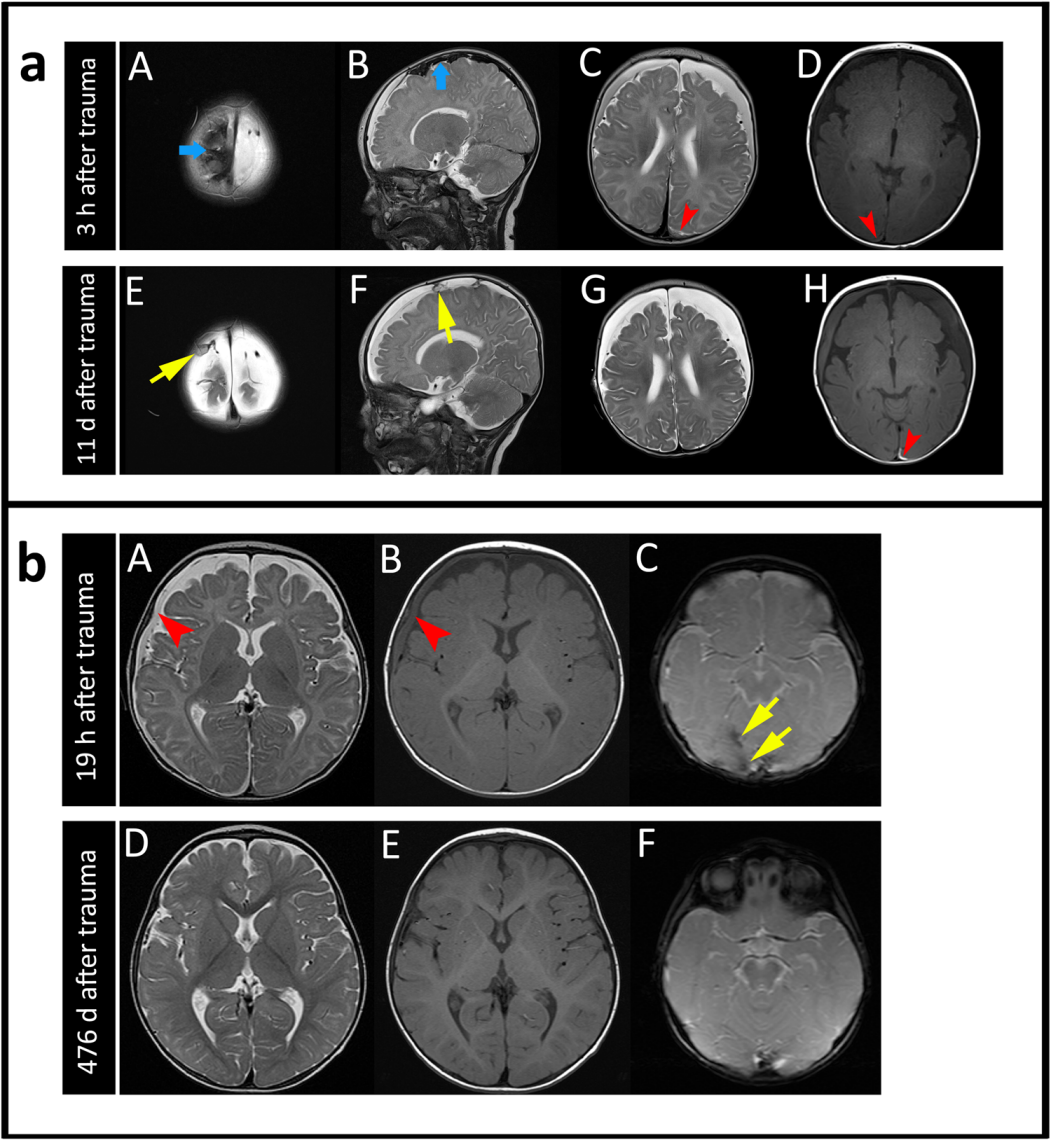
a MRI follow-up study of a 3-month-old boy showing a noticeable decrease of a subdural clot in a dominant SDHHy hete within 11 days. The figure shows axial T2w imaging proximal to the vertex (A *+* E), sagittal T2w imaging at the right hemisphere (B *+* F), axial T2w imaging (C *+* G), and axial T1w imaging (D *+* H). The detectable subdural blood clot (blue arrows, A *+* B) at the right parietal convexity 3 h after trauma decreased noticeably in a follow-up MRI scan 11 days after trauma. Instead, a tadpole sign at the right parietal convexity became visible (yellow arrows, E *+* F). Axial T2w imaging (C) and axial T1w imaging (D *+* H) showed an SDHHy hete with subdural sediment dorsally of the occipital lobes 3 h and 11 days after trauma (red arrowheads, C *+* D *+* H). b MRI scans of a 5-month-old girl at the time of the first scan showed complete regression of an SDHHy homo within 476 days. Initial MRI 19 h after trauma with axial T2w (A), T1w (B), and C T2*w imaging (C) showed a dominant SDHHy homo mainly at the right parietal convexity (red arrowheads), widened subdural spaces filled with fluid in both frontal regions, and small amounts of tentorial sediment in T2*w imaging (C) (yellow arrows). In a follow-up MRI 476 days after trauma no SDC was detectable in T2w (D), T1w (E), and T2*w imaging (F). Even widened subdural spaces and tentorial sediment in T2*w images (F) have disappeared
